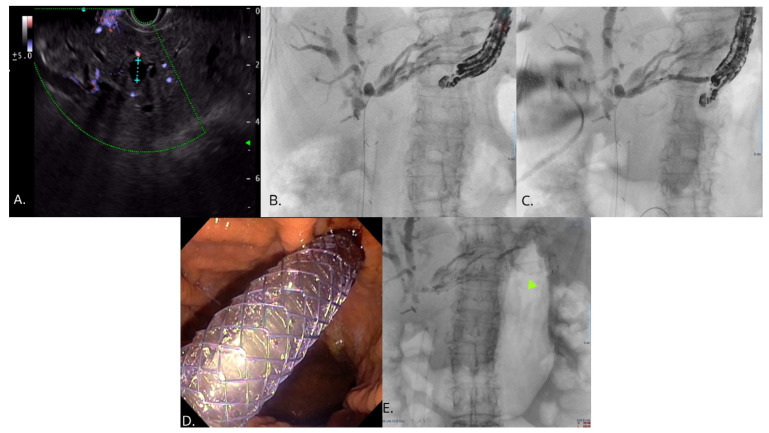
Hepaticogastrostomy steps. (A) Ultrasound view of the liver parenchyma. The application of color doppler allows the recognition of the dilated intrahepatic biliary ducts (within the caliper). (B) Under EUS guidance, a dilated intrahepatic biliary duct in segment III is accessed by puncture through the gastric wall, followed by contrast injection to visualize the biliary tree. A guidewire is then advanced under fluoroscopic view. A duodenal SEMS was previously placed to overcome a neoplastic stenosis. (C) The newly created fistulous tract is dilated with a dilation balloon. (D) A partially covered metallic stent is then released, connecting the right intrahepatic biliary system with the gastric lumen. (E) Final fluoroscopic view of the stent, as highlighted by the green arrow.
